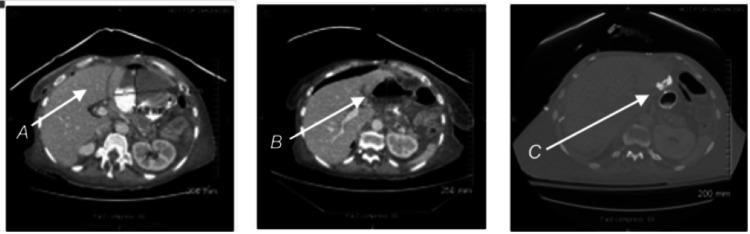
Multiple follow-up CT scans: A: Free contrast outside the lumen. B: Abscess formation. C: Endo-VAC and stent in place Arrow A indicates the area of free contrast outside the lumen, highlighting the site of the leak; Arrow B points to the formation of an abscess, showing the accumulation of fluid in the abdominal cavity; Arrow C marks the position of the Endo-VAC and stent in place, demonstrating the intervention used to manage the leak.
